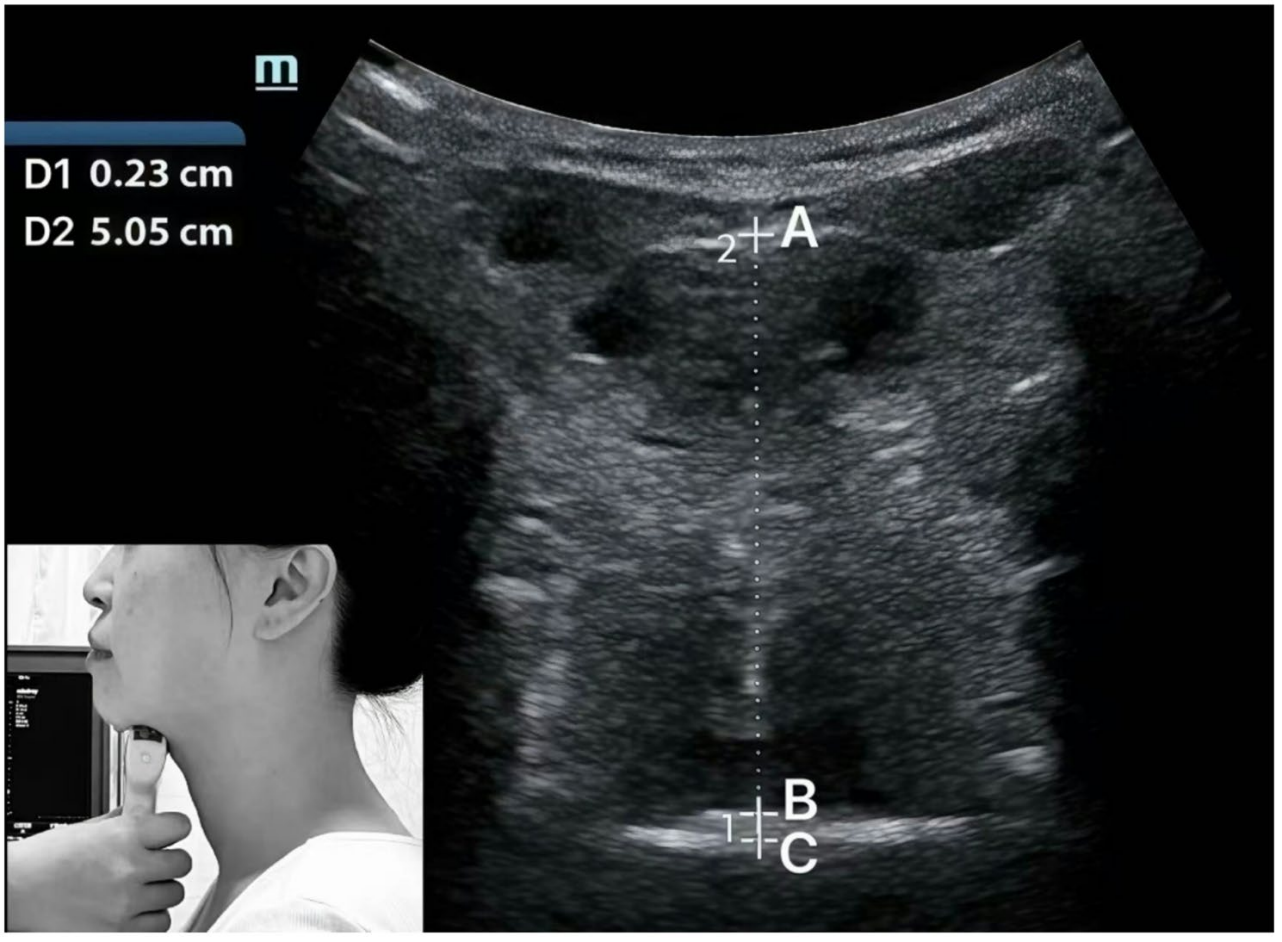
Measurements on the ultrasound image of tongue-palate distance and tongue thickness. D1 = BC: Tongue-palate distance, D2 = AB: Tongue thickness
